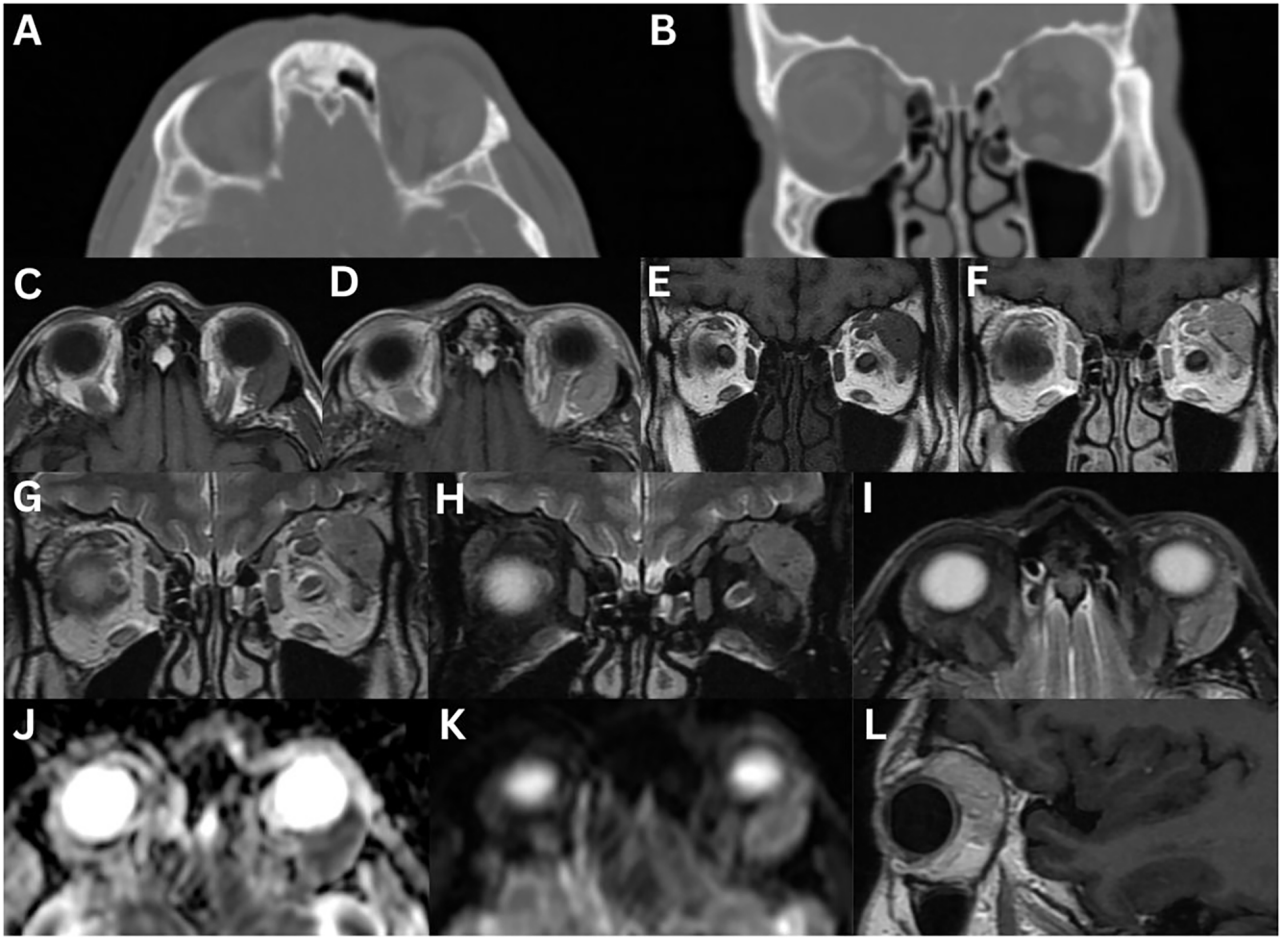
IgG4-related ophthalmic disease involving the left lacrimal gland. CT axial (A) and coronal (B) demonstrates no evidence of bony changes. MRI T1-weighted FLAIR axial (C) and coronal (E), contrast-enhanced FLAIR axial (D) and coronal (F), T2-weighted coronal (G), fat-suppressed coronal (H) and axial (I), and T1-weighted sagittal contrast-enhanced FLAIR (L) sequences demonstrate an isolated solitary enlargement of the left lacrimal gland. The lesion has a well-defined outline, with minimal vascularity increase in the affected portion of the lacrimal gland. Mild enhancement is observed following contrast administration. The gland is isointense on T1- and T2-weighted imaging relative to the adjacent extraocular muscles. There is evidence of significant restricted diffusion on axial ADC (J) and DWI (K).
